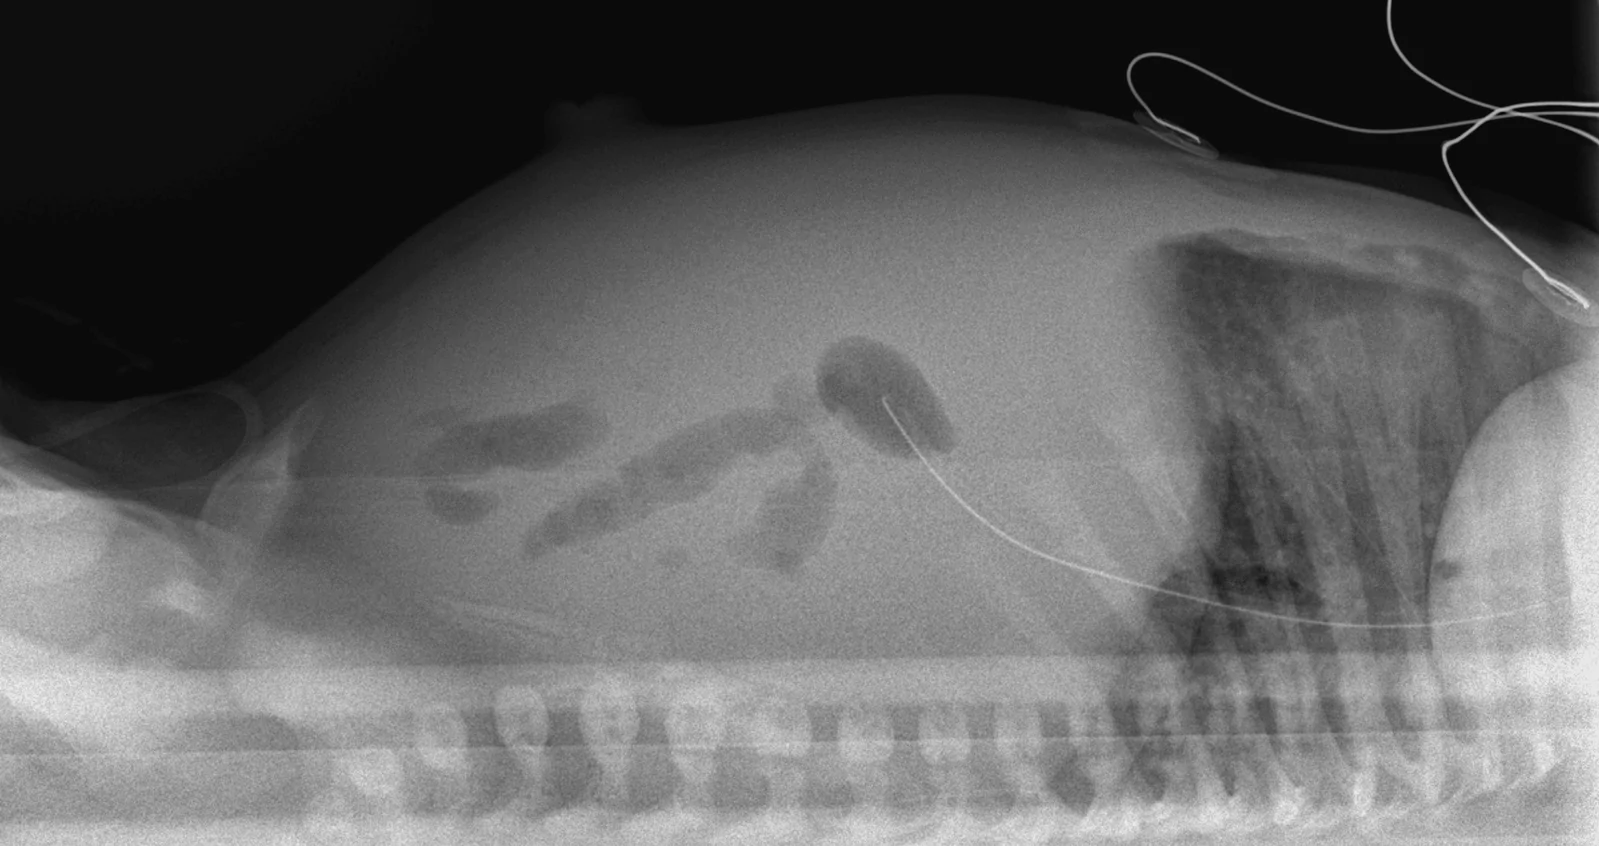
Lateral abdominal radiograph showing little visible air in bowel loops.
